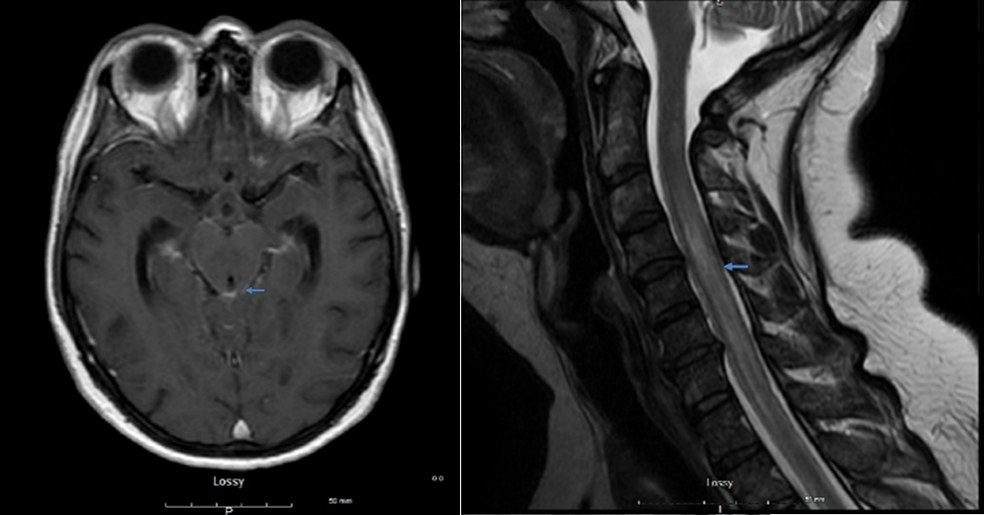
MRI brain and cervical spine showing leptomeningeal enhancement (shown by arrows) at the time of presentation
